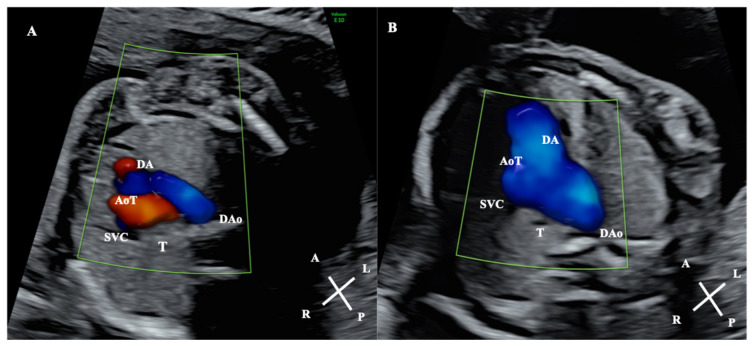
The standard 3VT view with color Doppler demonstrates the fetal thorax in a transverse plane. An incorrect Doppler angle, positioned perpendicular to the direction of flow, may create a misleading appearance of critical aortic obstruction by suggesting retrograde flow in the transverse aortic arch (AoT). From left to right: ductal arch (*DA*), AoT with apparent retrograde flow, and superior vena cava (SVC) (A). When the same image is evaluated using an appropriate Doppler angle, a normal 3VT view is observed, with antegrade, laminar flow in both the AoT and *DA*, directed posteriorly toward the descending aorta (DAo) (B).
